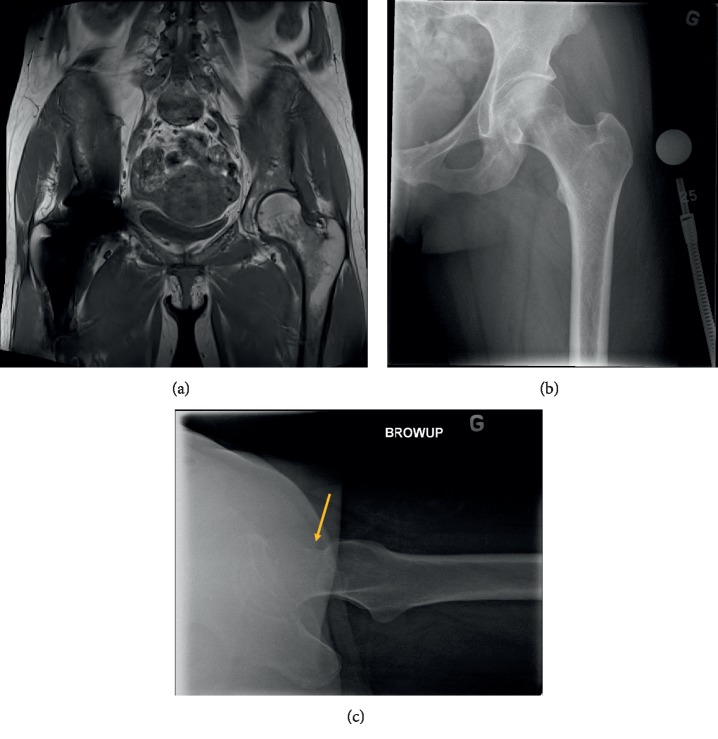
(a) Pelvic MRI showing bone marrow edema of the left hip on the compression side. (b) AP and (c) profile follow-up X-ray of the left hip showing a minimally displaced complete femoral neck fracture (arrow).
